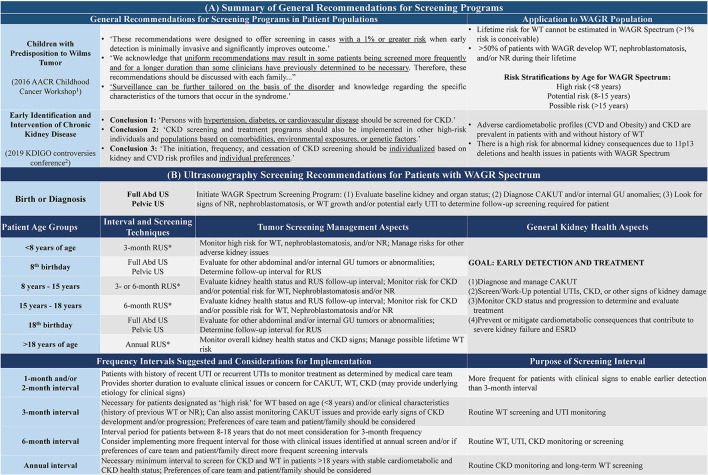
The WAGR spectrum screening program. All patients with WAGR Spectrum should receive routine renal ultrasonography (RUS) to monitor for kidney health throughout their lifetime. The principles underlying the recommendations developed for monitoring Wilms Tumor (WT) and Chronic Kidney Disease (CKD) with application to patients with WAGR are described in (A); recommendation groups included the ^1^American Association for Childhood Cancer Research recommendations (3) and ^2^Kidney Disease: Improving Global Outcomes (KDIGO) Work Group (39). (B) Provides recommendations for the age groups and suggestions for specific interval periods that could be applied for the RUS screening program. These intervals are based on the patient's age and potential risk—below the age of 8 years, all patients should be considered at risk for WT development and receive appropriate monitoring through RUS every 3 months (*unless more frequent indicated by detection or suspicion for renal abnormality). After the age of 8 years, consideration for frequency of follow-up should be determined in combination with clinical history, current status, and discussion between care team and patient/family to determine the best individualized course. All patients should at least receive annual RUS to monitor for CKD risk. Additional imaging such as full abdominal U/S and pelvic U/S are warranted at certain periods as the patient ages to monitor the status of other internal organs and GU anomalies.
